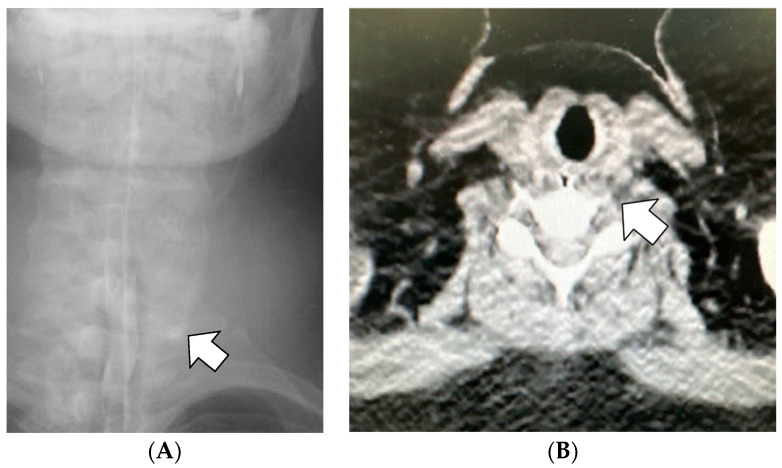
Fluoroscopy and contrast computed tomography (CT) images on postoperative day 1. (A) The arrow indicates contrast accumulation observed on the left side of the neck. (B) A CT scan performed immediately afterward does not show any contrast accumulation and increased focal fatty tissue density is observed. The arrow in (B) points to the same location as in (A).
